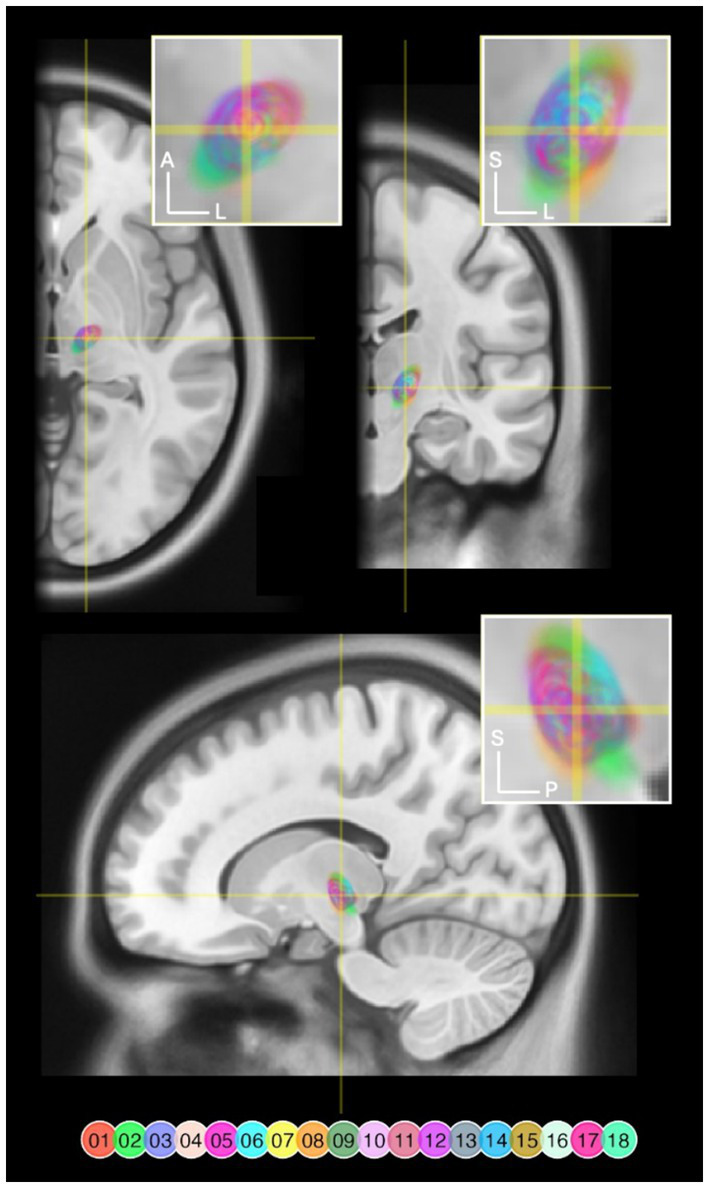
VTAs rendered and overlaid on a MNI T1 Magnetic Resonance Image (MRI) in axial (*z* = 141), coronal (*y* = 232), and sagittal (*x* = 170) slices. Right-sided VTAs were mirrored so that these are projected onto the left hemisphere. The crosshairs indicate where the slices intersect. The yellow rectangles delineate the target areas of each magnification inset. VTA pixels are color coded by lead number and flattened along the axis that is not visible in that specific view. VTA pixels are transparent to allow overlapping areas to blend. A, anterior; L, left; S, superior; P, posterior.
